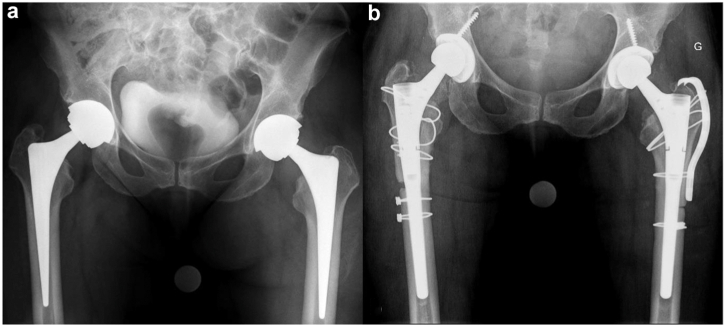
Anteroposterior pelvis radiograph (a) prior to and (b) 5 months after bilateral revision for systemic metal toxicity and bilateral periprosthetic joint infection.
